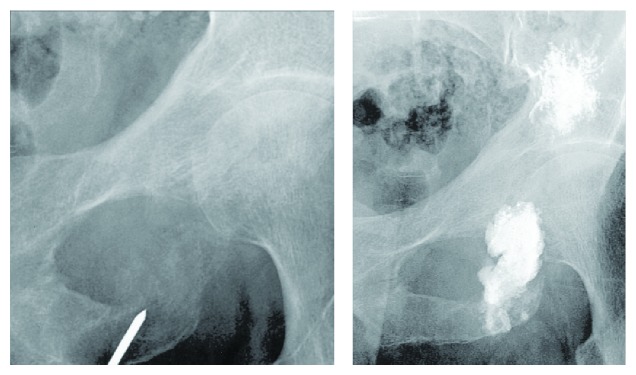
Intra- and postoperative radiographies of an acetabuloplasty.
